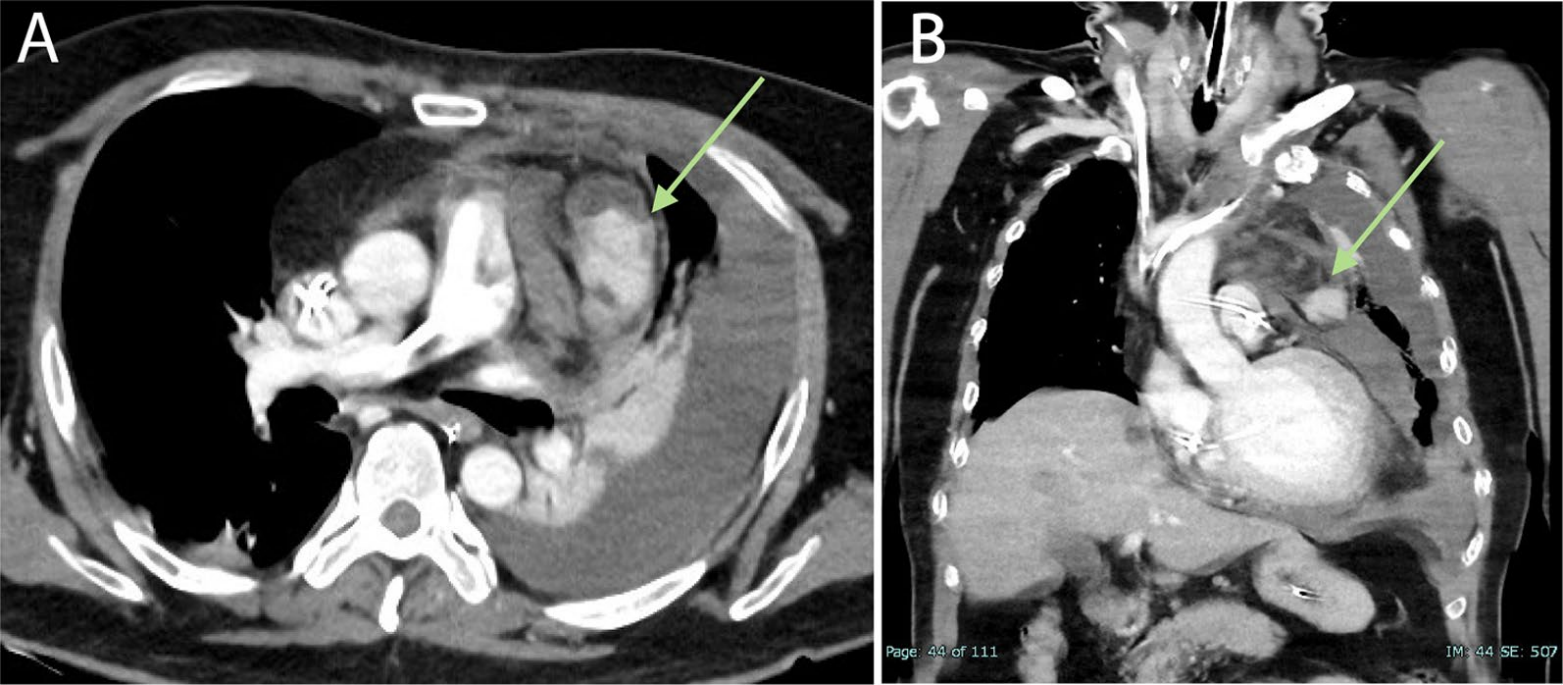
Preoperative images of enhanced computed tomography. A Axial view and B coronal view. Venous hemorrhage from the thymus gland was noted (green arrow)
